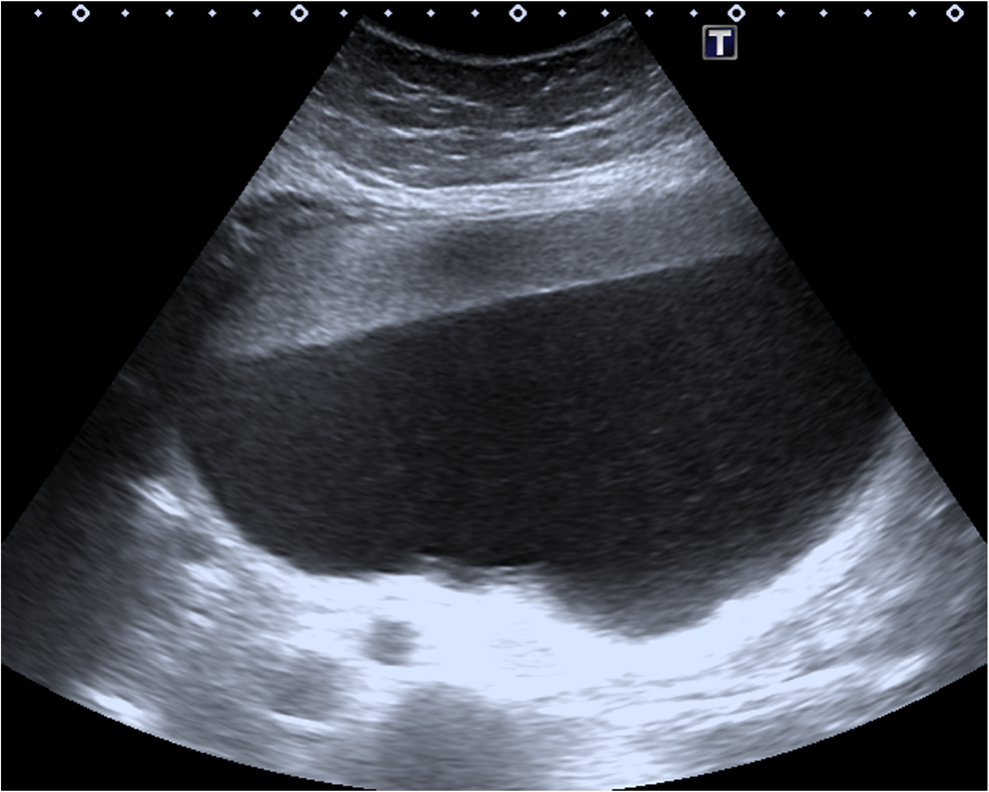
Transverse sonogram of an 18-year-old female with fat-fluid level in a mature cystic teratoma. Supernatant hyperechoic sebum forms a layer with dependent hypoechoic aqueous fluid
